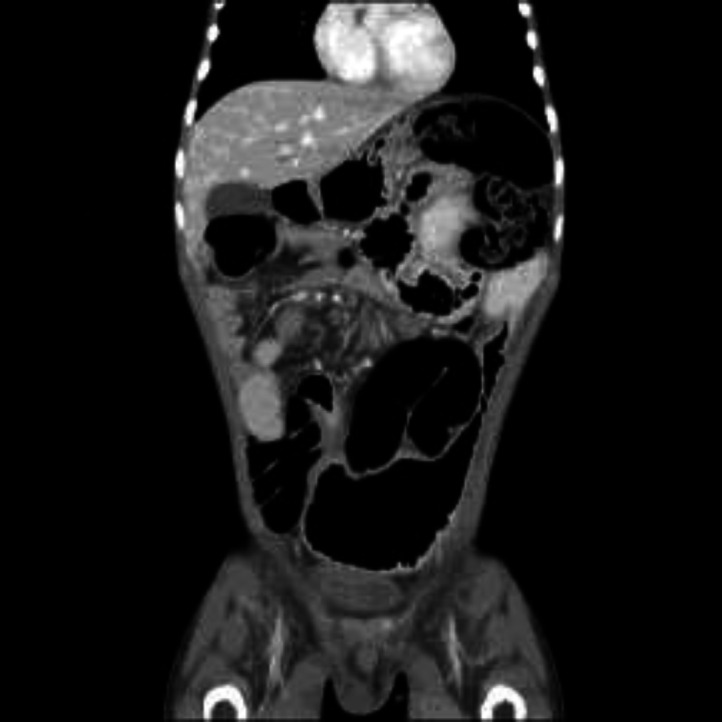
Coronal reformatted CT image showing alternate thickening of the wall of the terminal ileum (white arrow) and adjacent normal wall thickness (blue arrow), suggesting skip lesions in the terminal ileum
